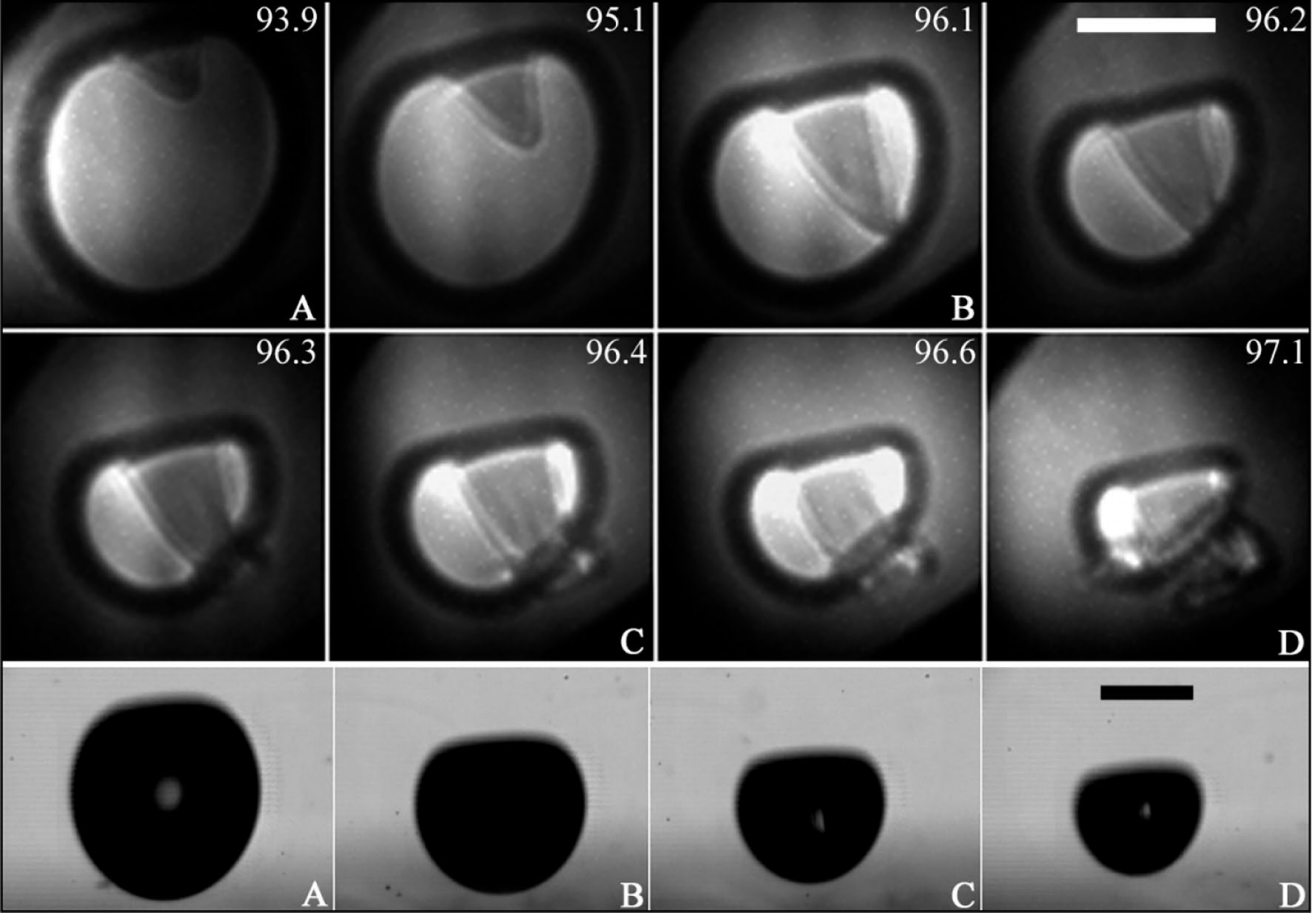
X-ray holographic images for a bubble with a stand-off parameter $$\gamma =1.28\pm 0.03$$ located at a radial distance $$\alpha _r = 2.0 \pm 0.4$$ from the border of the glass disc. The shadowgraph images in the bottom row are $$90^{\circ }$$ tilted and the jet is pointing to the camera. Corresponding frames are marked with the same capital letters. Here, the optical frames are advanced 690 ns with respect to the x-ray holograms. The scale bars represent $$250\,{\upmu \text {m}}$$ and the numbers time in $$\upmu {s}$$. Multimedia view: (Video Fig. 7.avi)
